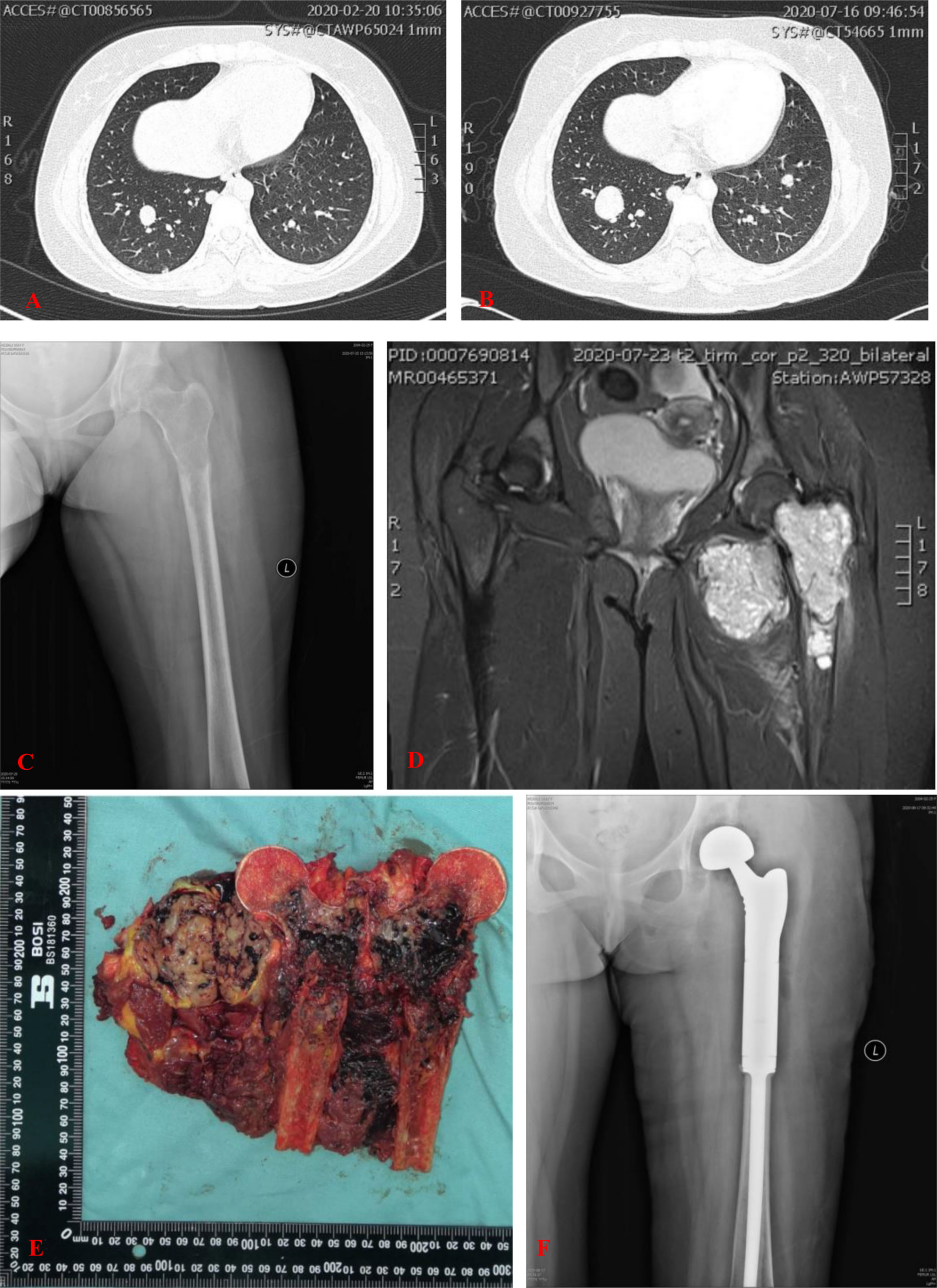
(A) CT showed multiple nodules in both lungs. (B) CT showed part of nodules in both lungs was enlarged 5 months later. (C) Plain radiograph of the left femur showed an aggravated lytic lesion in the upper segment of the left femur. (D) MRI showed the intramedullary soft tissue mass was enlarged. Intramuscular soft tissue mass in close proximity to the medial side of the lesser trochanter had no obvious changes. (E) Grossly, the tumor was centrically located in the proximal femur. Cut section of the proximal femur showed a black cut surface involving the intramedullary space with areas of hemorrhage and necrosis. The adjacent extraosseous mass had a yellowish-brown fleshy cut surface measured 6.4 × 4.8 cm in axial diameter. (F) Postoperative anterior posterior radiographs of the left femur demonstrated the endoprosthetic reconstruction of the proximal femur and hip joint.
